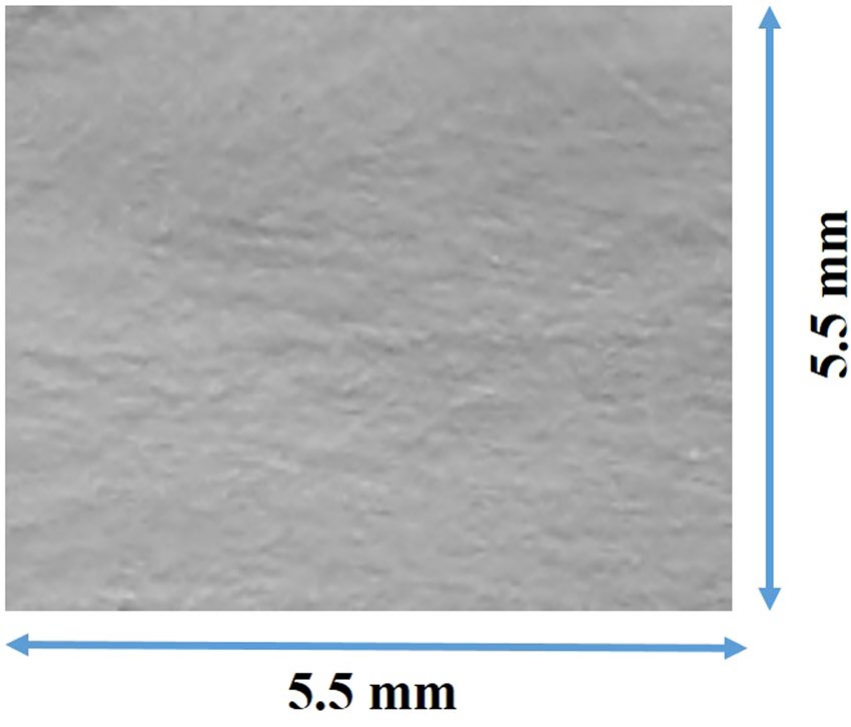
X-ray topographic image of an as-grown Cd_0.9_Zn_0.1_Te_0.98_Se_0.02_ sample. Exposed area: ~5.5 × 5.5 mm^2^.
